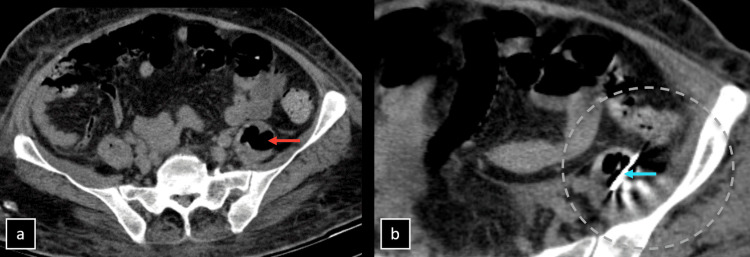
Non-contrast-enhanced computed tomography (CT). Non-contrast-enhanced CT, axial sections show a (a) pre-procedural image displaying the left psoas abscess (orange arrow) and (b) precise placement of the tip of an 18-gauge spinal needle within the left psoas abscess (cyan arrow), highlighting the technical precision of the CT-guided catheter drainage procedure.
